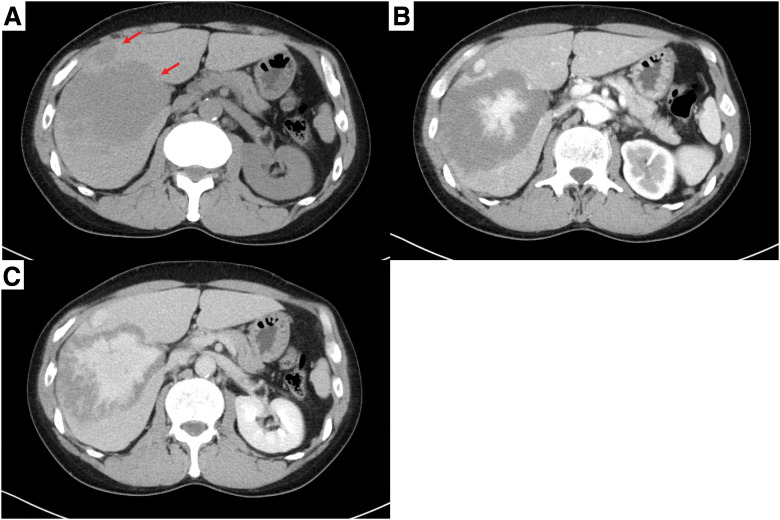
Abdominal CT images. Plain CT revealed multiple homogeneous low-density lesions (red arrows) (A). Dynamic contrast-enhanced CT demonstrated gradual enhancement extending from the center toward the periphery in the arterial phase (B), with persistent enhancement in the equilibrium phase (C).
